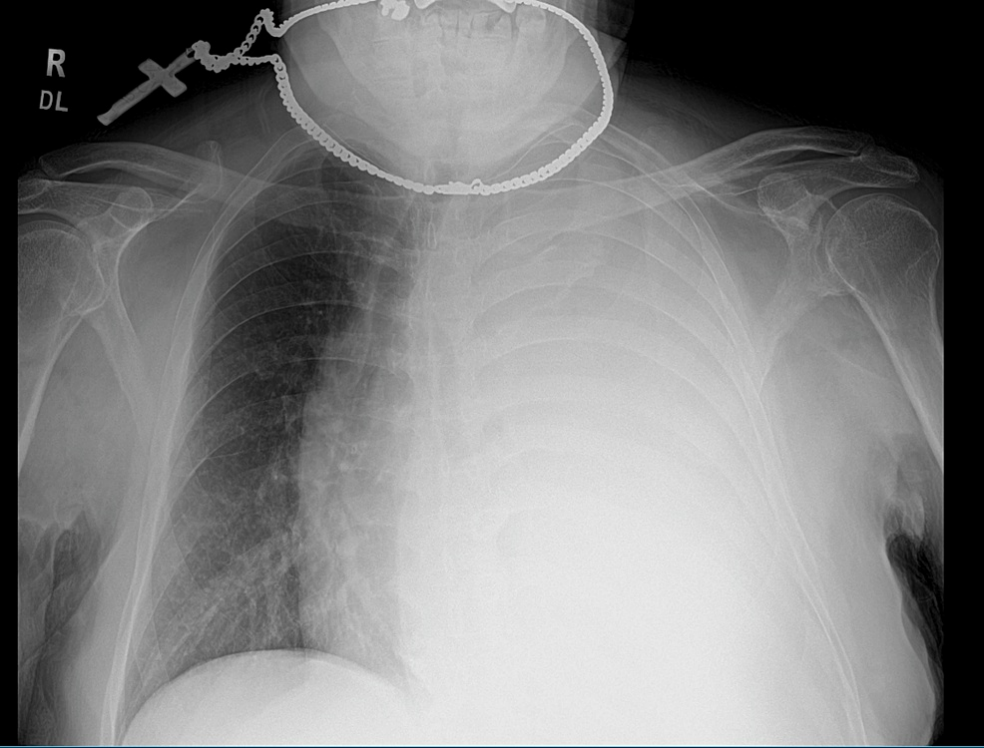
Chest X-ray showing complete opacification of the left lung most likely due to left-side pleural effusion.
